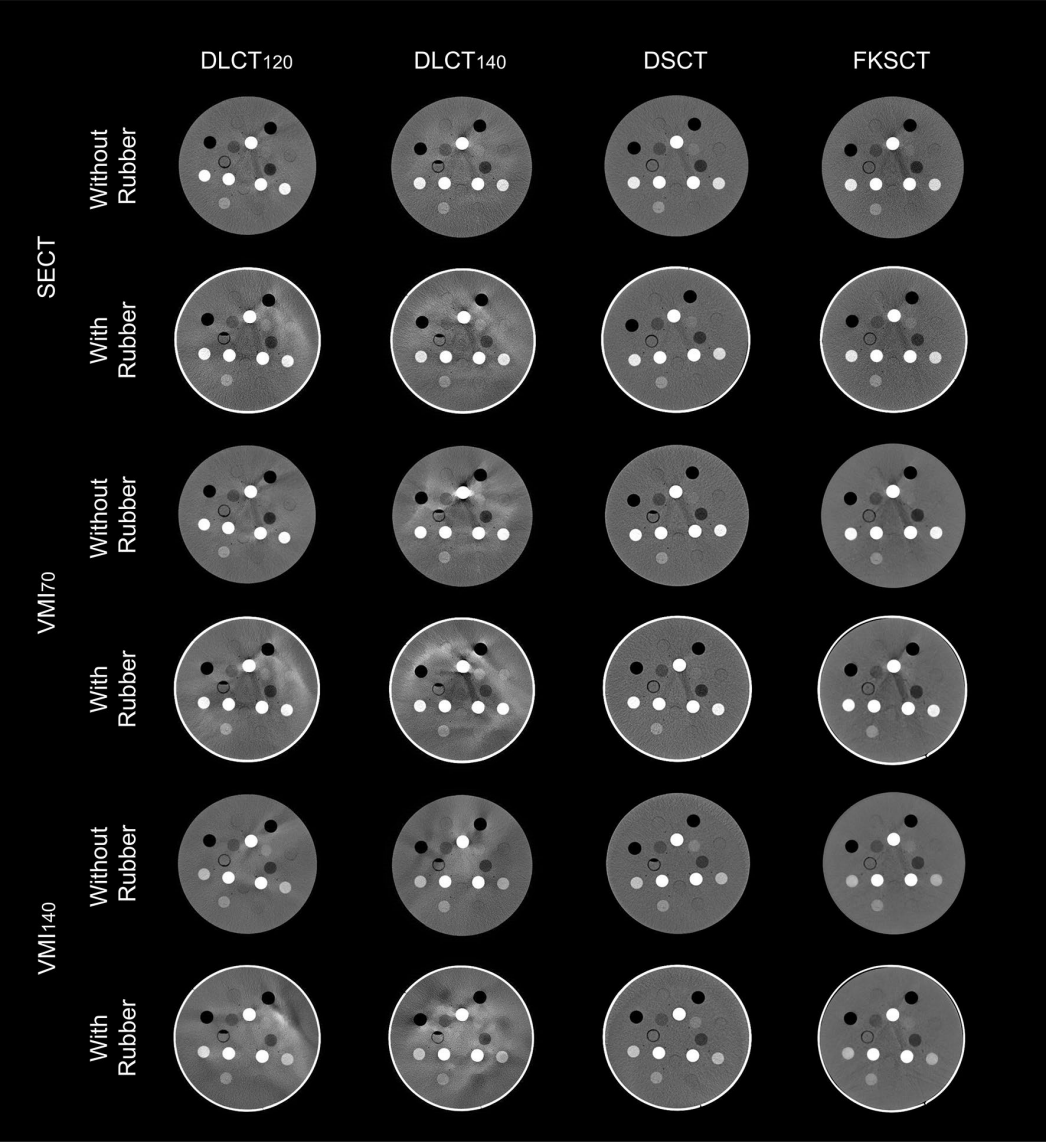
Single-energy computed tomography (SECT) images and virtual monochromatic images at 70 keV (VMI_70_) and 140 keV (VMI_140_) acquired using dual-layer CT with tube voltage of 120 (DLCT120) and 140 (DLCT140) kVp, dual-source CT (DSCT), and fast kilovoltage switching CT (FSKCT) scanners
